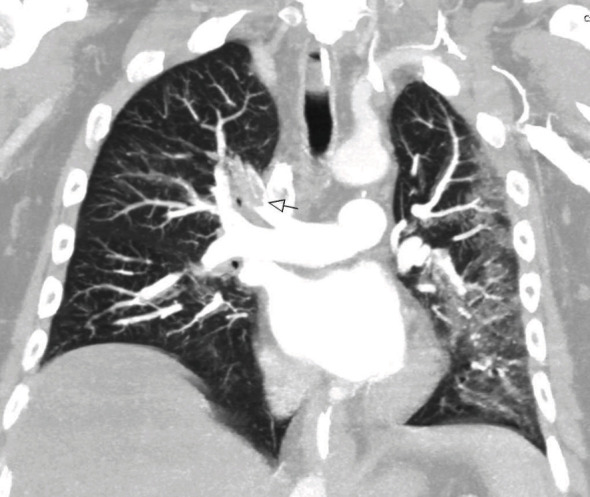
CT pulmonary angiogram performed on day 3 showing right-sided proximal segmental pulmonary embolism.
